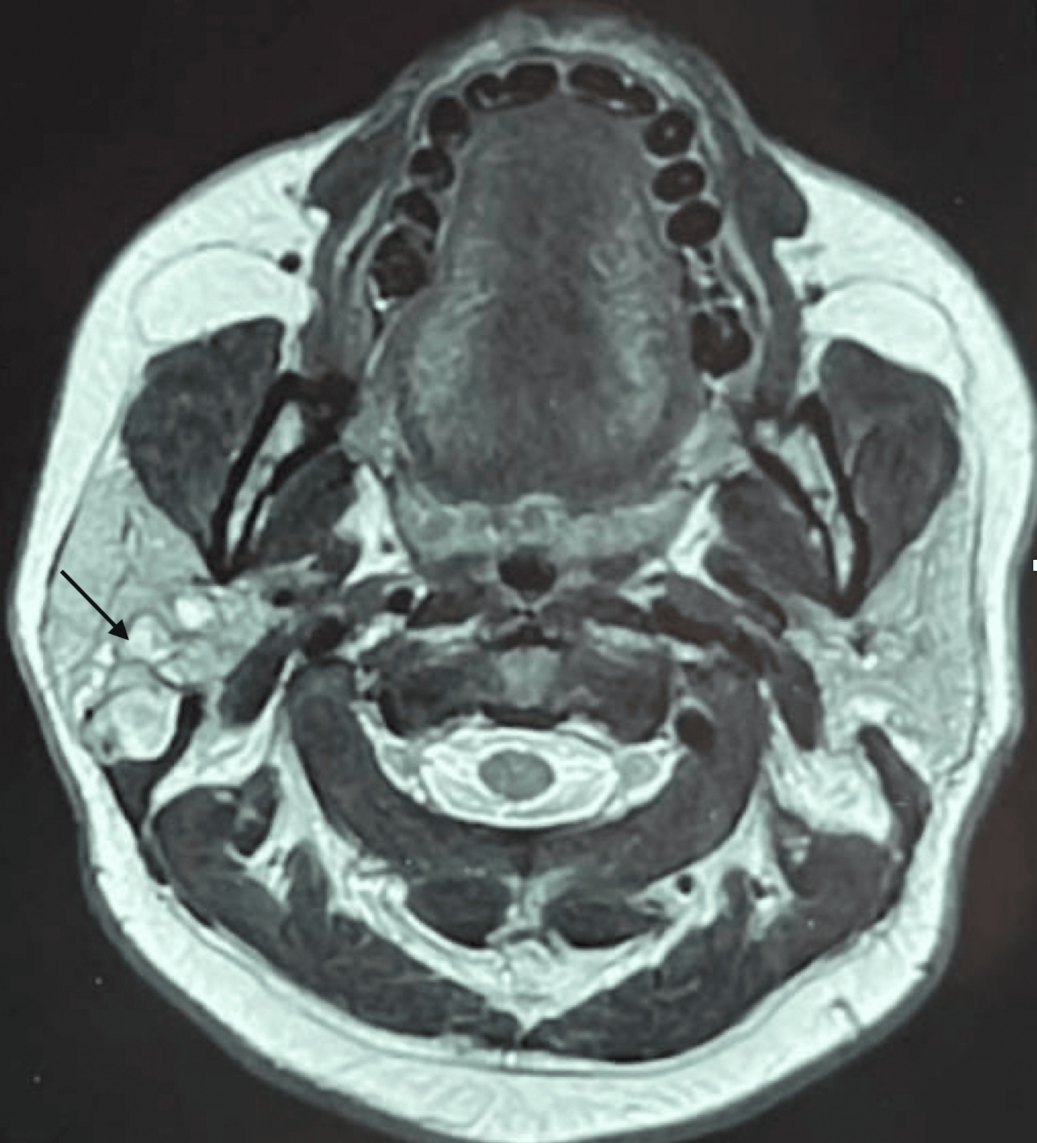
Axial MRI images showing a well-defined right parotid mass suggestive of a pleomorphic adenoma. The arrow indicates the parotid mass.
